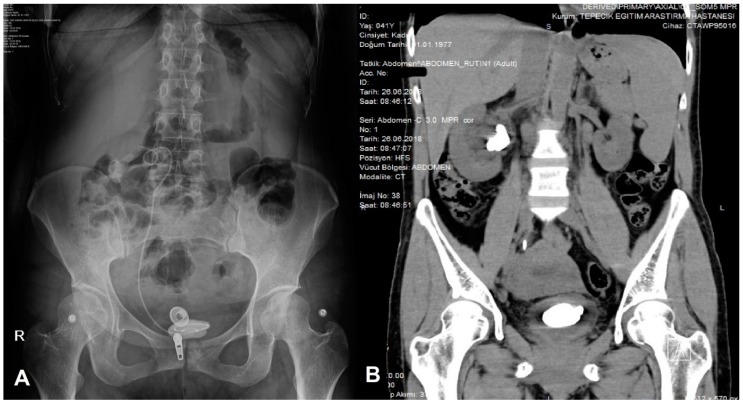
Heavily proximal and distal encrustation and stone formation of an FUS, which retained for 28 months. (A) KUB film; (B) non-contrast CT (computed tomography).
